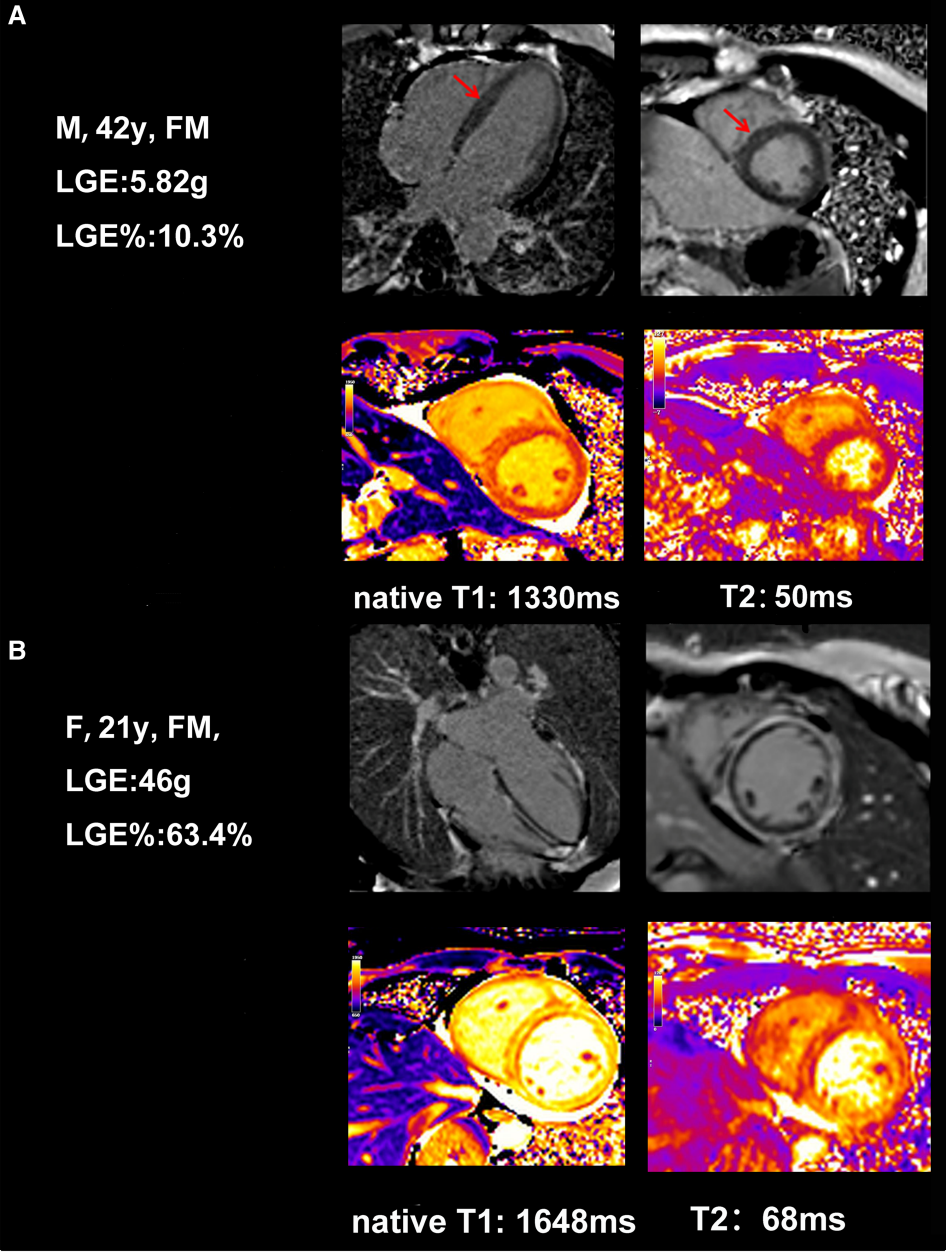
Example of typical cardiac MRI findings in a 42-year-old female FM patient with low LGE and a 21-year-old female FM patient with high LGE. (A) A 42-year-old woman with CMR short-axis and long-axis view with septal LGE (arrows) and global native T1, T2. (B) A 21-year-old woman with CMR short-axis and long-axis view with diffuse and a large amount of LGE in the intermediolateral segments, and global native T1, T2.
